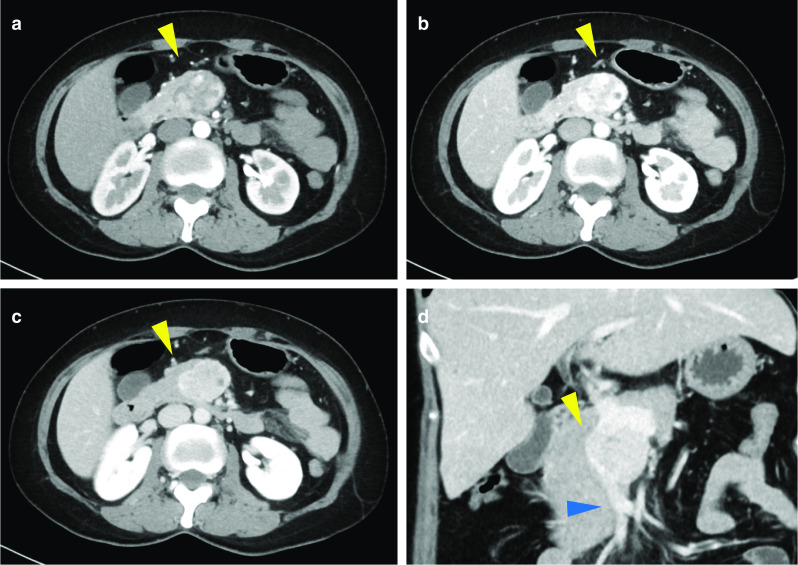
Dynamic-CT scan (a early arterial phase, b late arterial phase, c and d portal phase). Dynamic-CT scan revealing a hypovascularized tumor (yellow arrowhead) invading the superior mesenteric vein (blue arrowhead)
